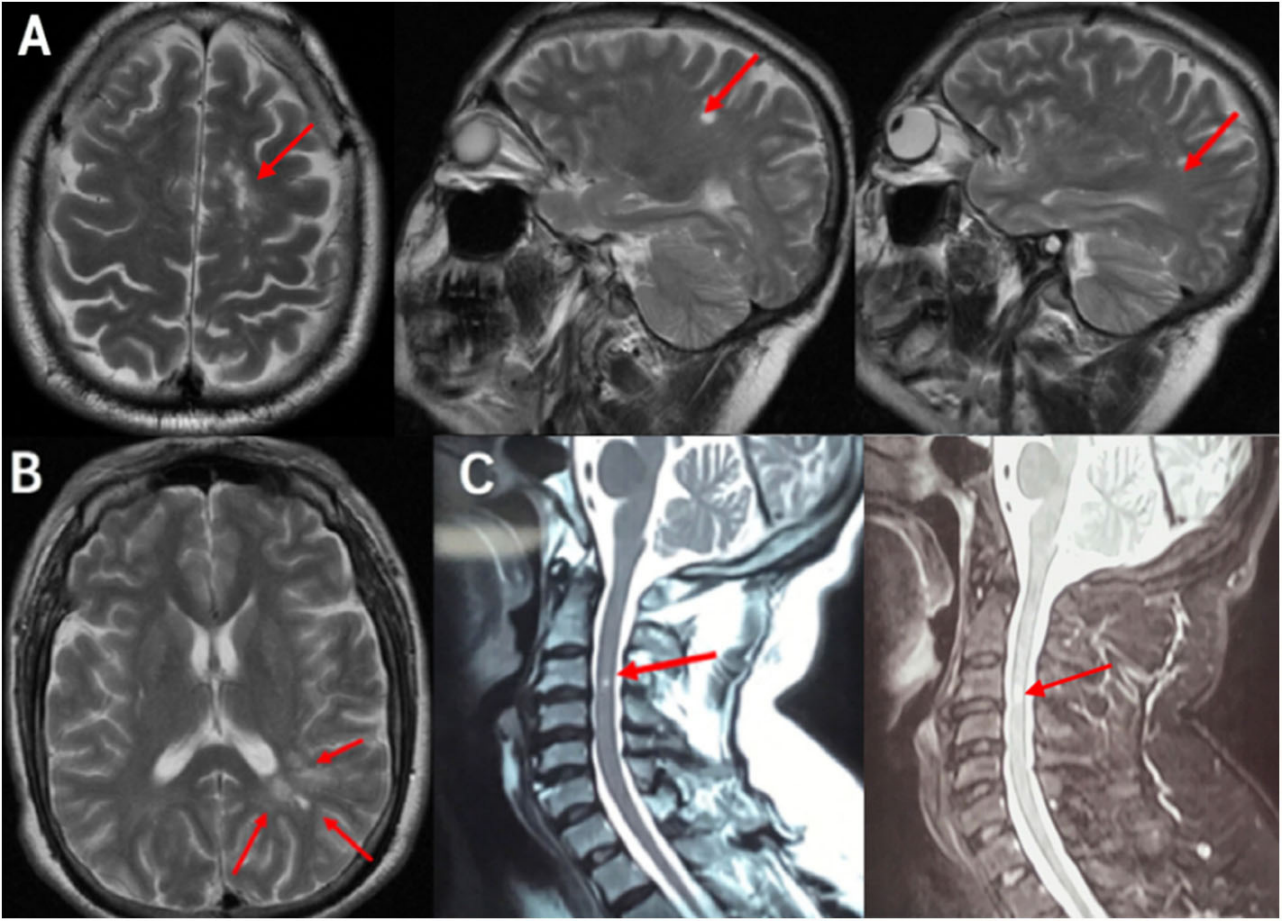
MRI scan demonstrated the DIS: (A) Cranial MRI T2 scans revealed three lesions in the cortex and juxtacortical lesions. (B) Cranial MRI T2 scans revealed multiple white-matter lesions in the periventricular lesion. (C) Cervical MRI scans revealed a cervical 3rd segment with hyperintensity signal in T2 and enhanced T2. Red arrows showed lesions, which was preliminary diagnosed demyelination. MRI, magnetic resonance imaging; DIS, dissemination of lesions in space.
